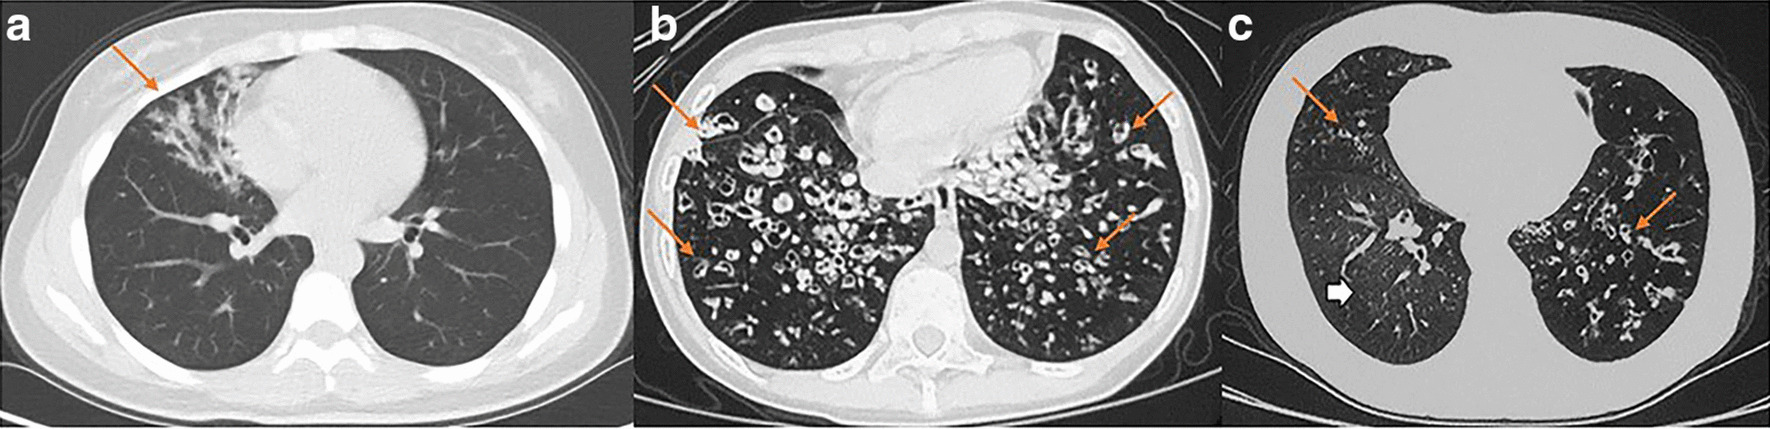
The bronchiectasis of patients by CT scan. a Bronchiectasis in the right middle lobe in patient 11. b Diffuse bronchiectasis in the right middle lobe, lingular segment, and both lower lobes in patient 14. c Bronchiectasis in the right middle lobe and left lower lobe (red arrows) in patient 20. In addition, air trapping was observed in his right lower lobe (white arrowhead)
